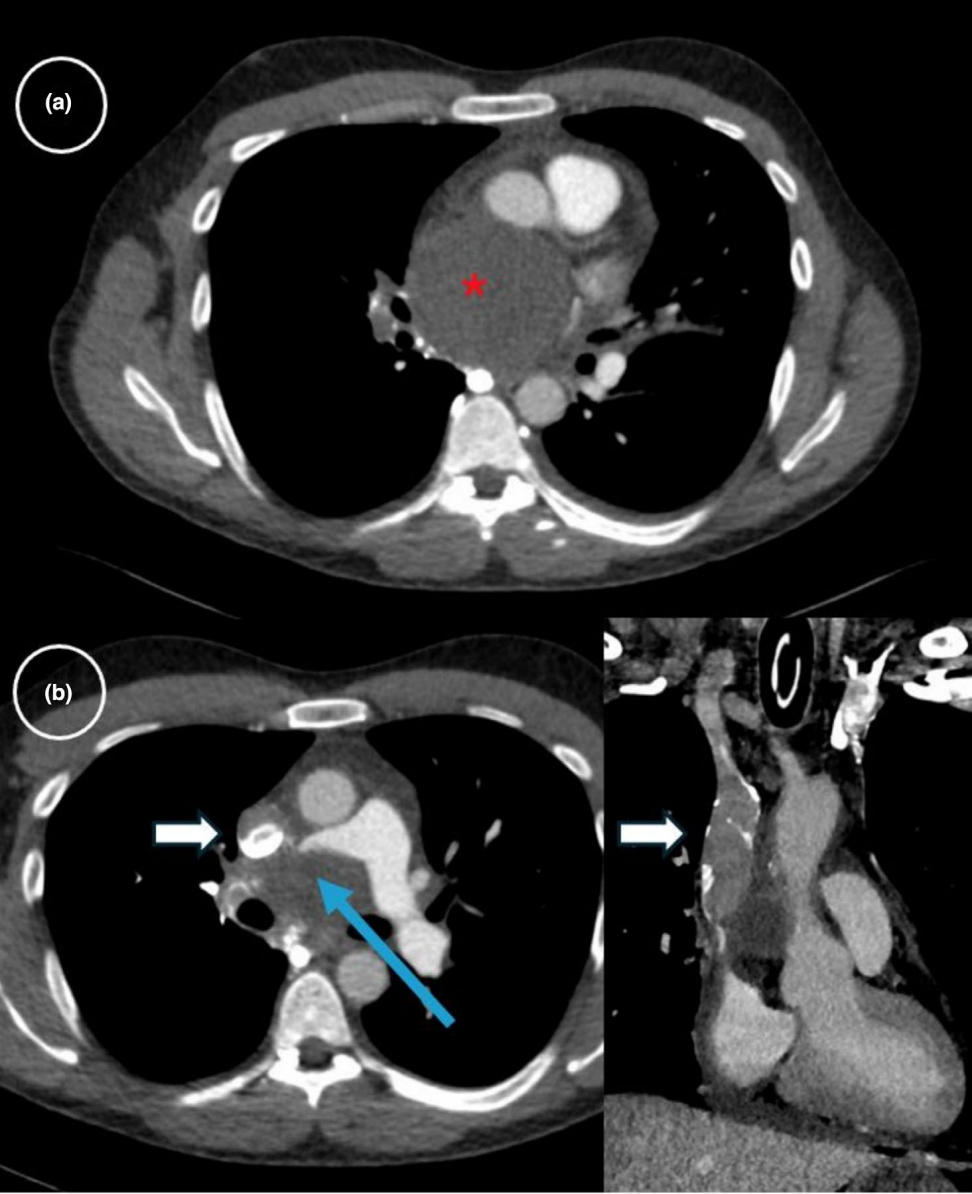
Contrast‐enhanced CT on admission showing (a) (*) a cystic mediastinal mass in the superior‐middle mediastinum, with (b) (white arrow) subocclusive thrombus in the superior vena cava and (blue arrow) near‐complete compression of the right pulmonary artery.
